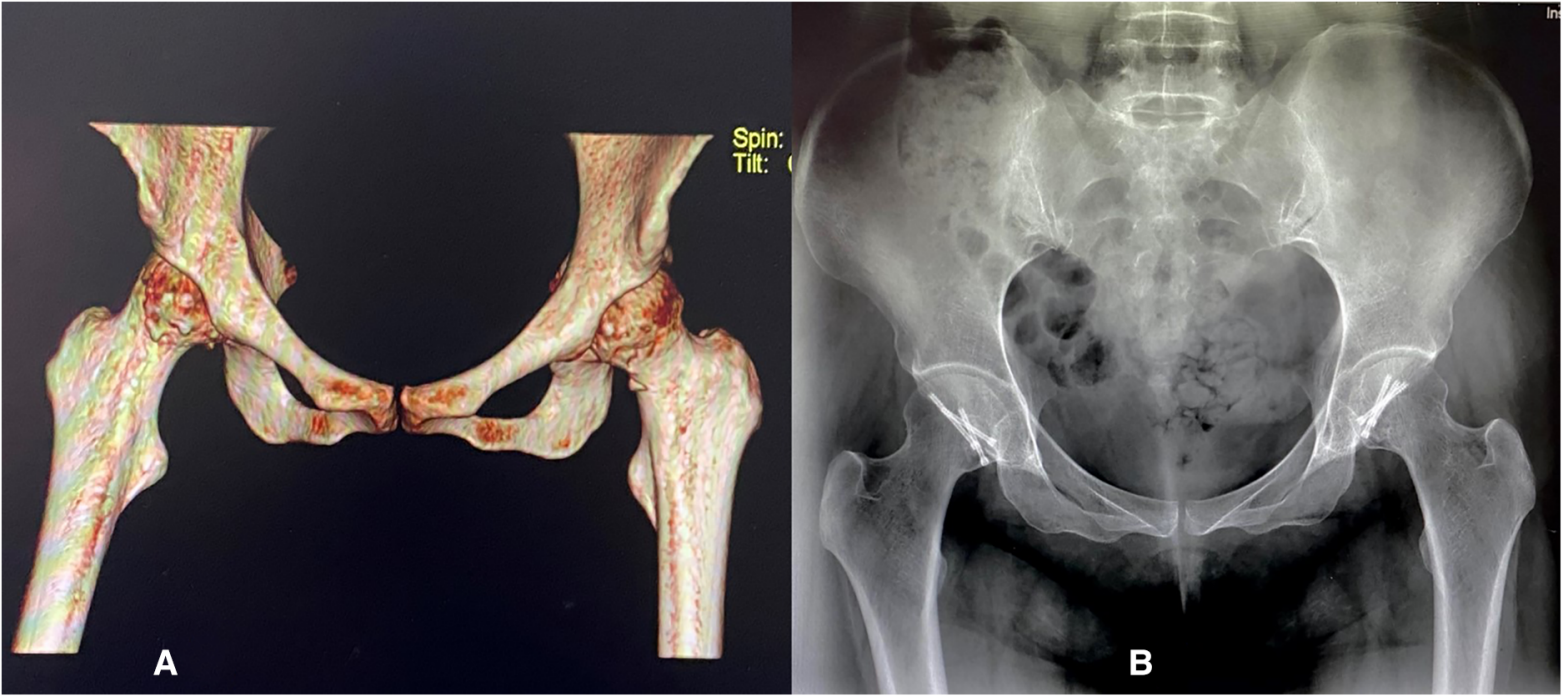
(A) Postoperative 3D CT and (B) X-ray anteroposterior (AP) images of the pelvis showing bilateral internal fixation in the femoral heads with three Herbert screws.
